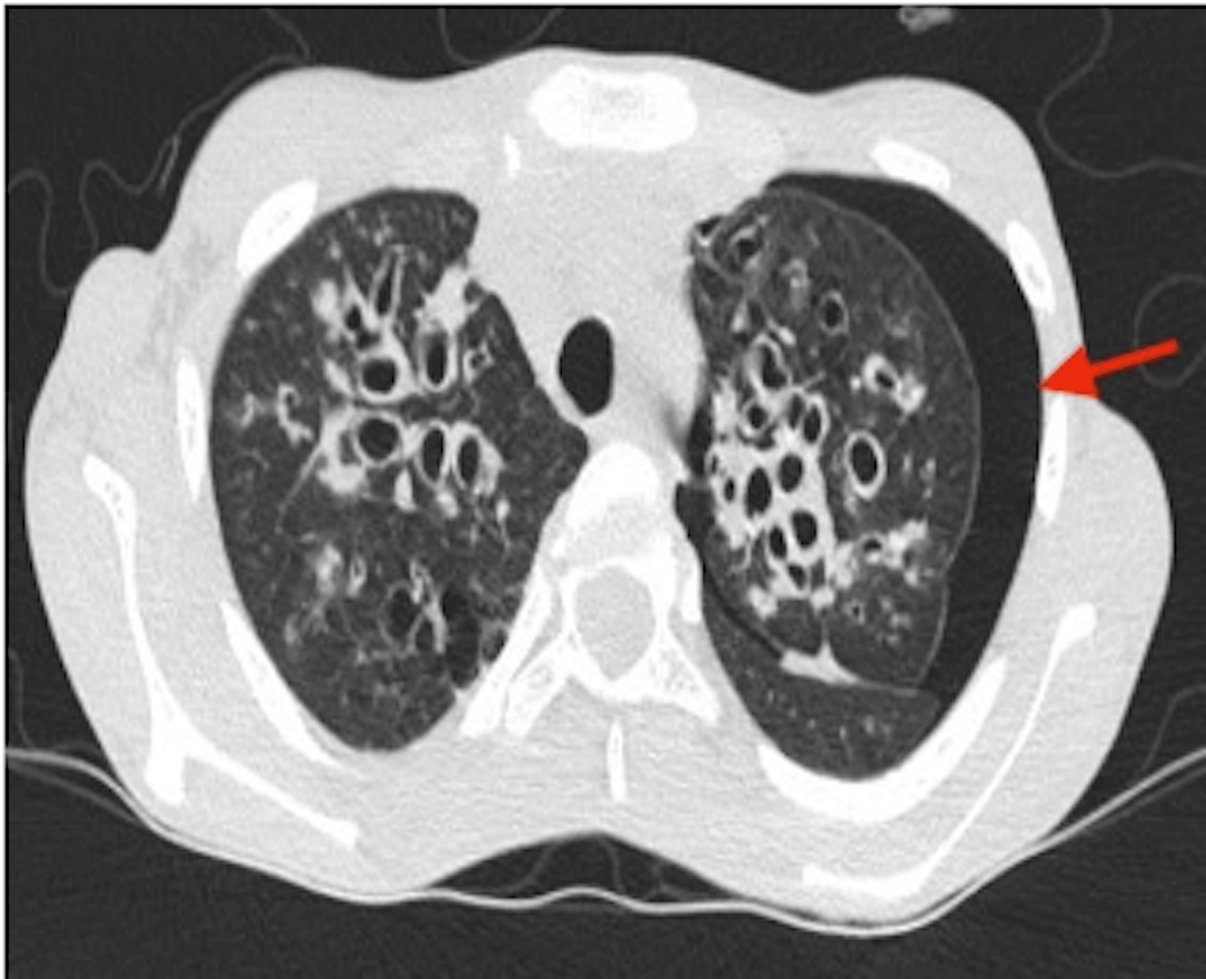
CT showing left-sided pneumothorax Red arrow: pneumothorax
